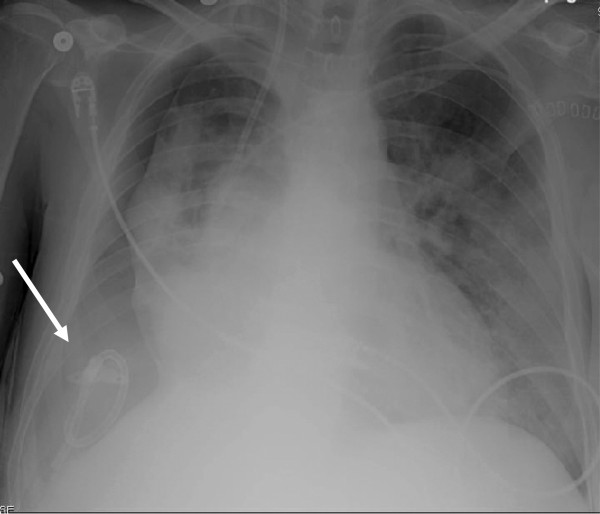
Chest x-ray post-OLT: Pneumothorax ex-vacuo. Arrow indicates location of chest tube.
